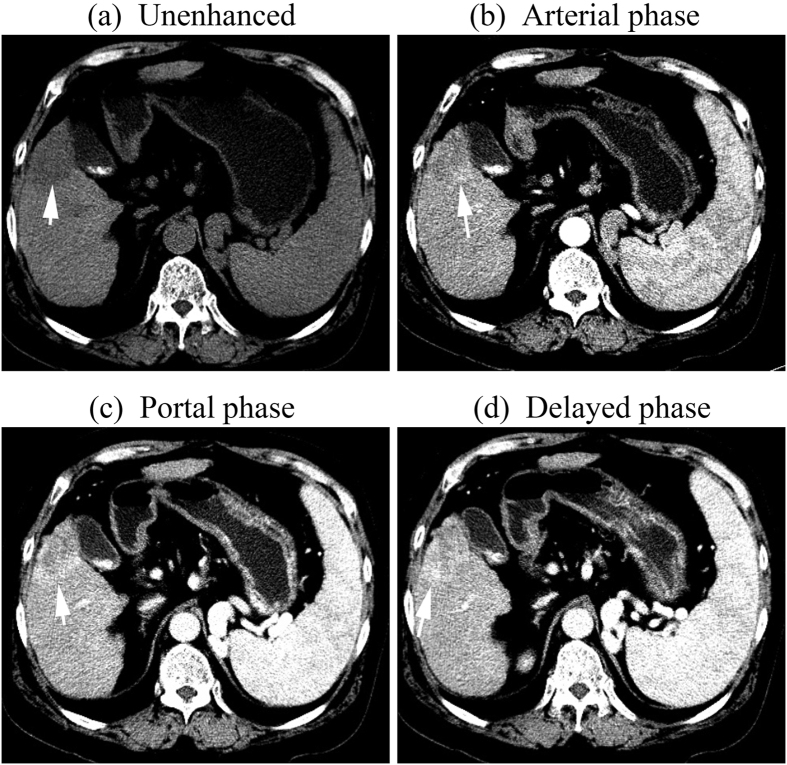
tif CT images of a 75-year-old man with a 3.8-cm –diameter ICC
